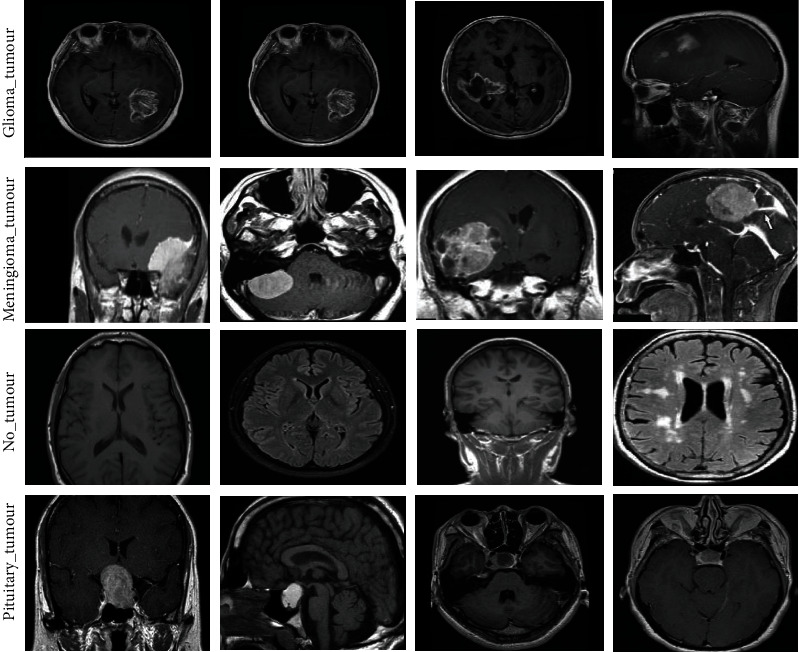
Samples of a dataset of an MRI of brain tumours. Source: (https://www.kaggle.com/sartajbhuvaji/brain-tumor-classification-mri).
